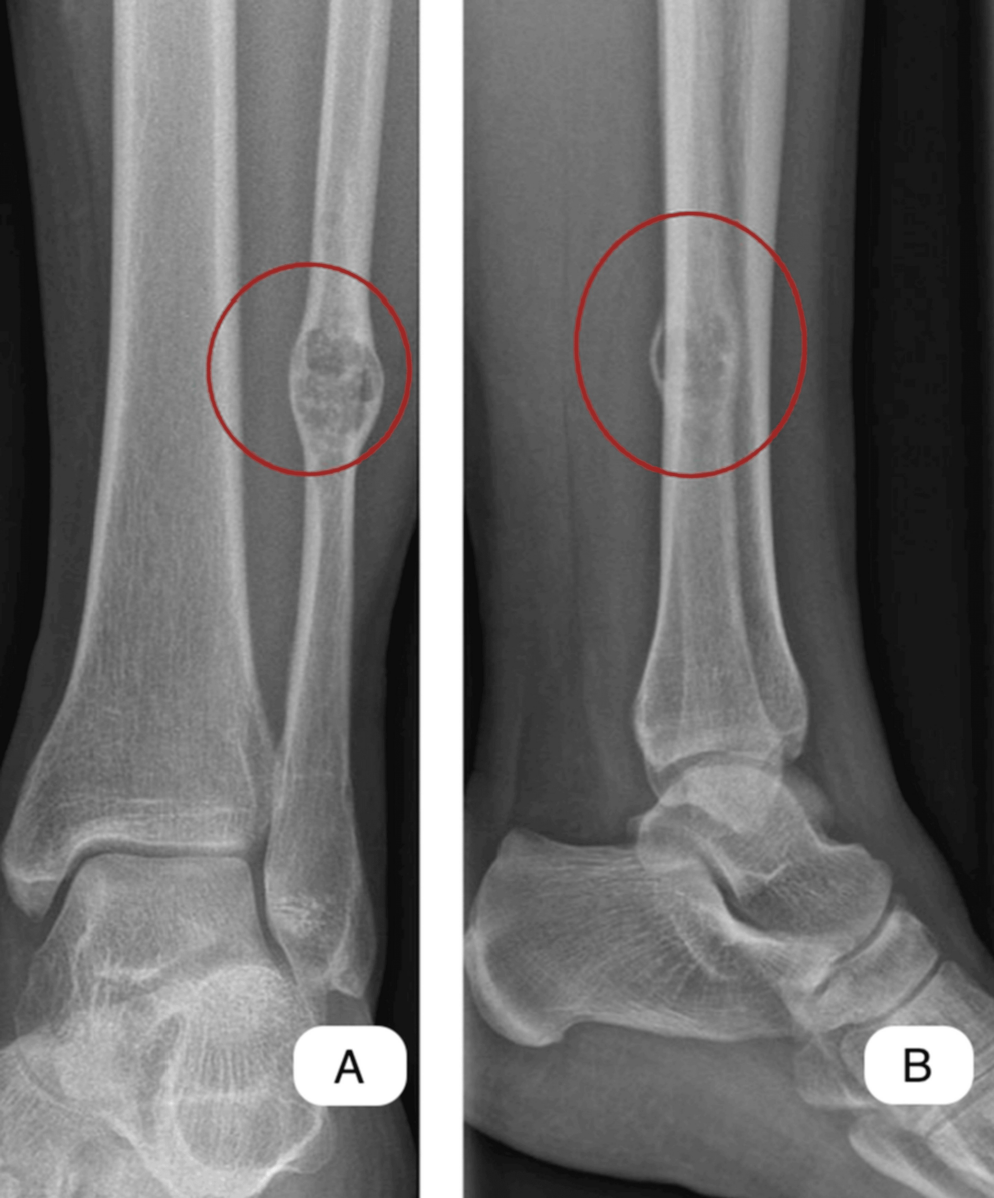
X-ray view of the distal 1/3 of the fibula shaft A - antero-posterior view B - lateral (profile) view
